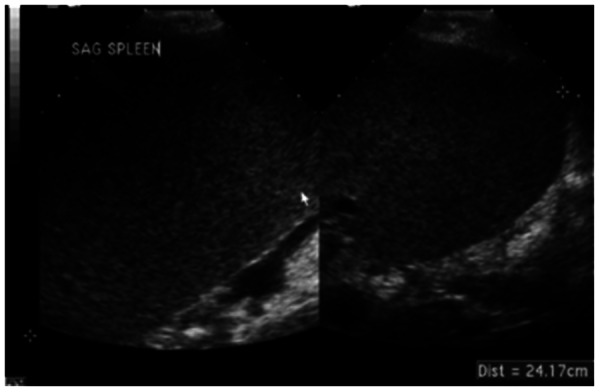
Ultrasound image of massive splenomegaly
